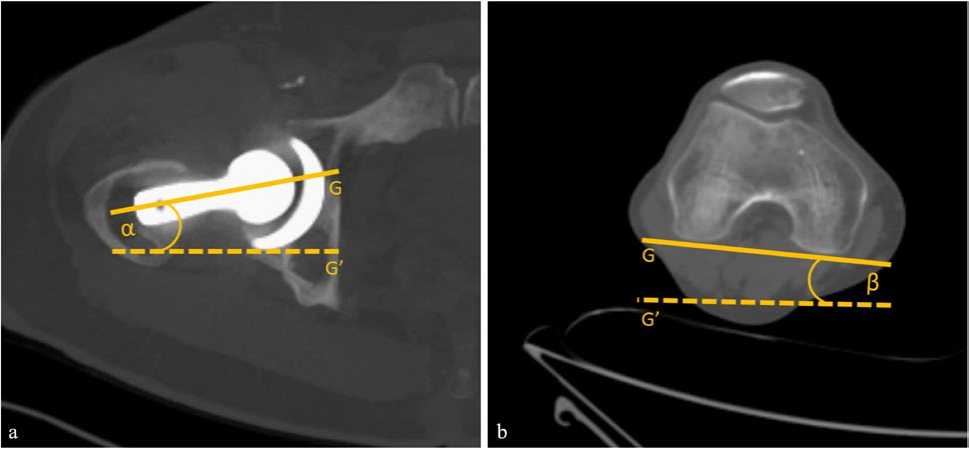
Calculation of femoral anteversion on axial CT images. The angle was defined by lines that were drawn through the prosthesis neck and the center of the prosthesis head (a) as well as through the posterior tangent connecting the most distal points of the femoral condyles (b). For calculating the femoral anteversion, two angle measurements were taken using a horizontal line (*G*′) as a fixed reference for the femoral neck and femoral condyle axis. The resulting angles were then added or subtracted, depending on whether they were oriented in the same or opposite direction
